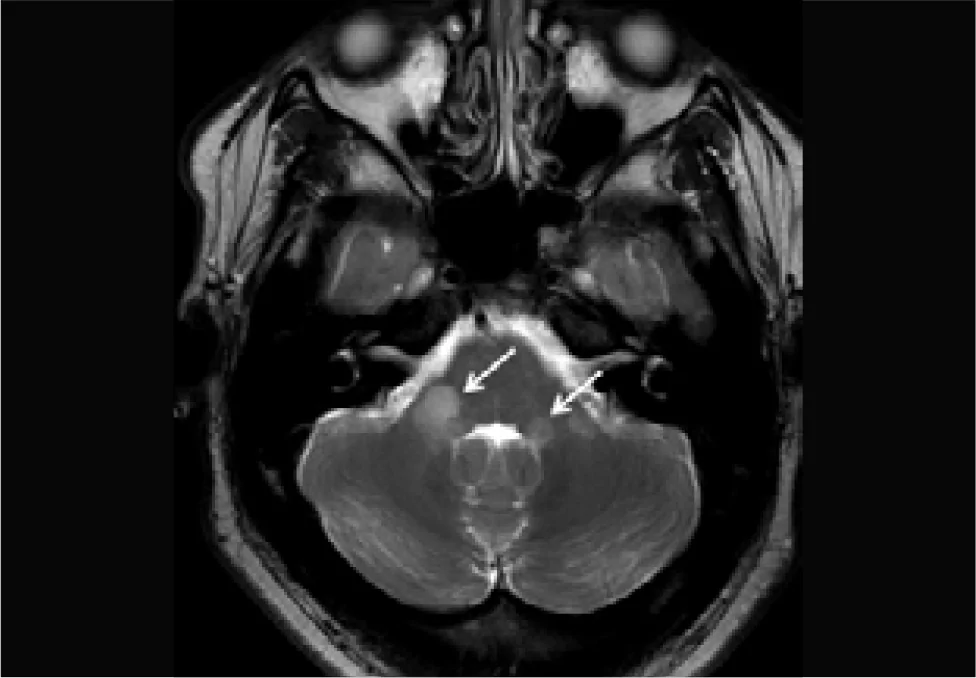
MRI of a 43-year-old woman presenting with ataxia. Axial T2WI showing
